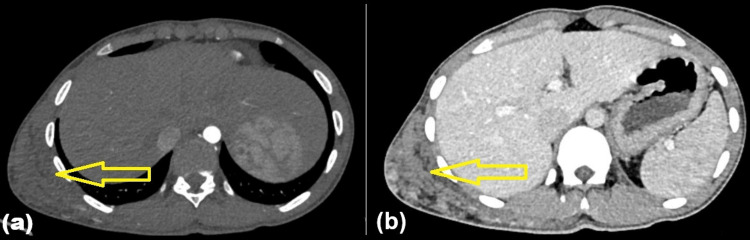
CECT scan of the chest, abdomen, and pelvis with angiography. (a) Axial CT angiography image, showing arterial supply to the chest wall lesion from multiple intercostal arteries. (b) Axial CECT scan, showing a large, ill-defined lobulated lesion involving the subcutaneous and muscular planes of the right posterior chest wall CT: computed tomography; CECT: contrast-enhanced computed tomography
